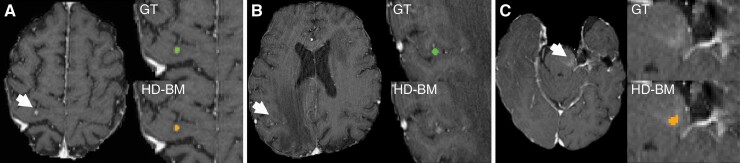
Example of true positive (A), false negative (B) and false positive (C) findings in the institutional/external dataset. Three example MRI studies with axial T1-weighted postcontrast images. (A) HD-BM (orange) shows accurate detection of BM (white arrow) in the right precentral gyrus comparable to the ground-truth (GT) segmentation (green). (B) Missed BM (green) were mostly small or associated with subtle contrast enhancement as shown here in the right parietal lobe (white arrow). (C) False positive findings (orange) were predominantly associated with vascular changes (white arrow; capillary telangiectasia).
